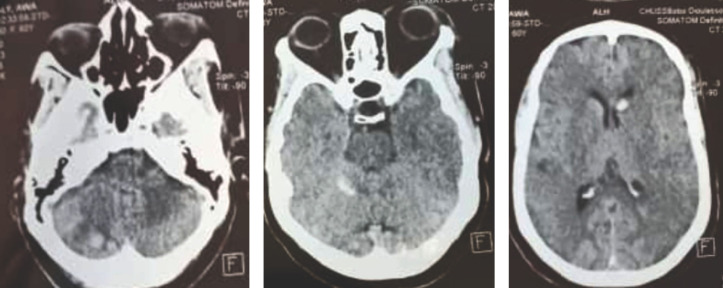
Tomodensitométrie cérébrale montrant de multiples saignements intracrâniens au niveau occipital, cérébelleux et ventriculaire Brain computed tomography showing multiple bleeds in occipital, cerebellar and ventricular regions
